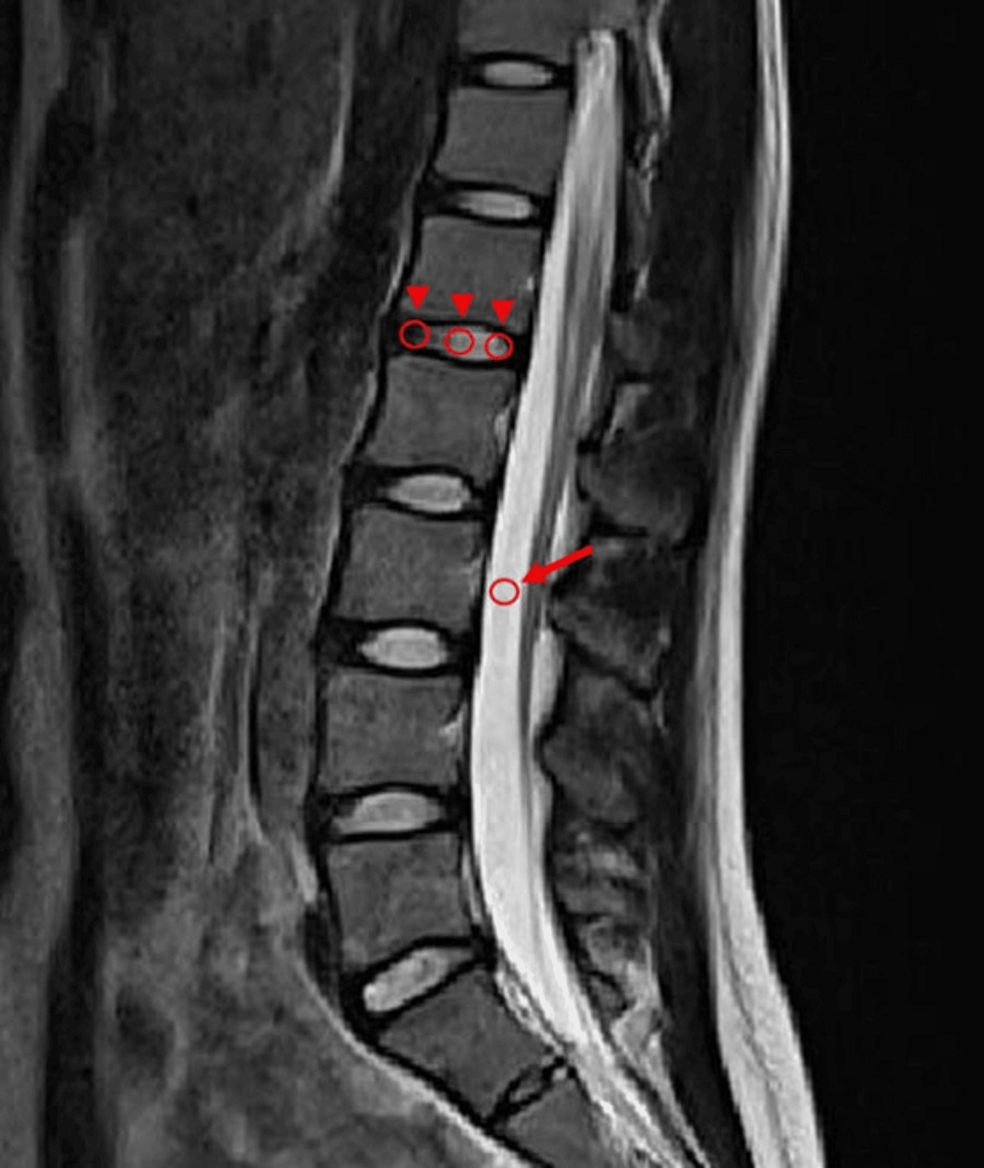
Representative ROI measurements of the disc and CSF signal intensities on T2-weighted midsagittal MRI images. Disc signal intensities were measured in three regions at the L1/2 disc (arrowhead) along with the ROI of CSF signal intensity (arrow). ROI, region of interest; CSF, cerebrospinal fluid; MRI, magnetic resonance imaging
